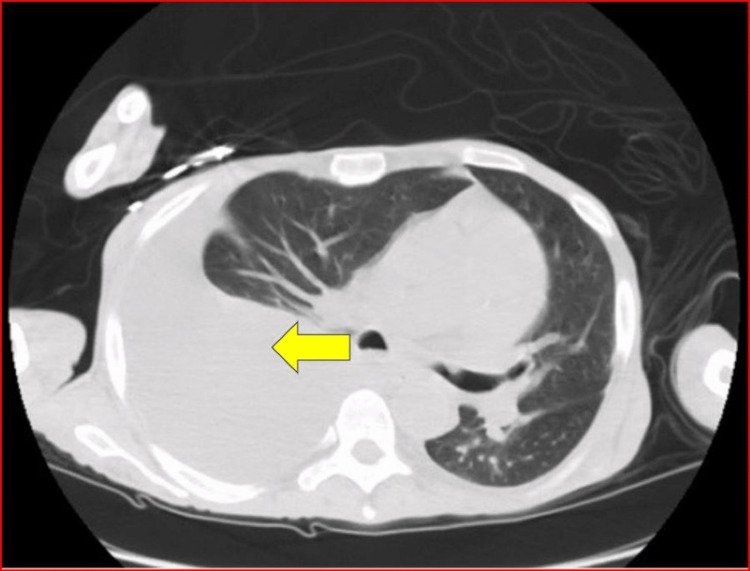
CT chest with right pleural effusion (yellow arrow).
